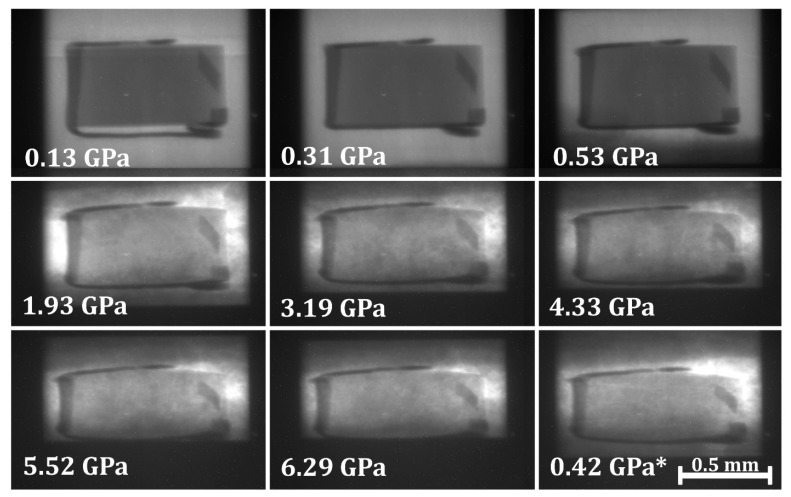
White-beam X-ray radiography images of α-Fe at increasing pressures and on decompression (noted with an ‘*’). The gold foil represented by the dark features represents the vertical and horizontal extent of the α-Fe sample.
